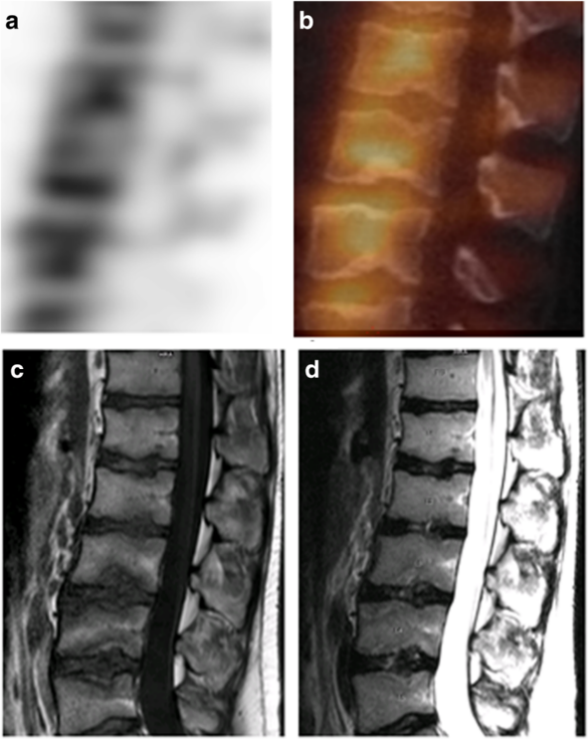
Twenty-year-old male with chronic longstanding lumbar pain with physical activities due to adolescent disk dysplasia. Bone scan shows heterogeneous uptake in the spine associated with endplates changes. Sagittal bone SPECT image of the lumbar spine (a) and fused SPECT/CT images (b) show heterogeneity of uptake in the vertebral bodies. Sagittal T1 (c) and T2 (d) weighted MR sequences showed straightening of the lumbar lordosis, endplate disc desiccation seen from T12-L1 through L5-S1, Schmorl's nodes at multiple levels, and early Modic type 1 endplate degenerative changes.
